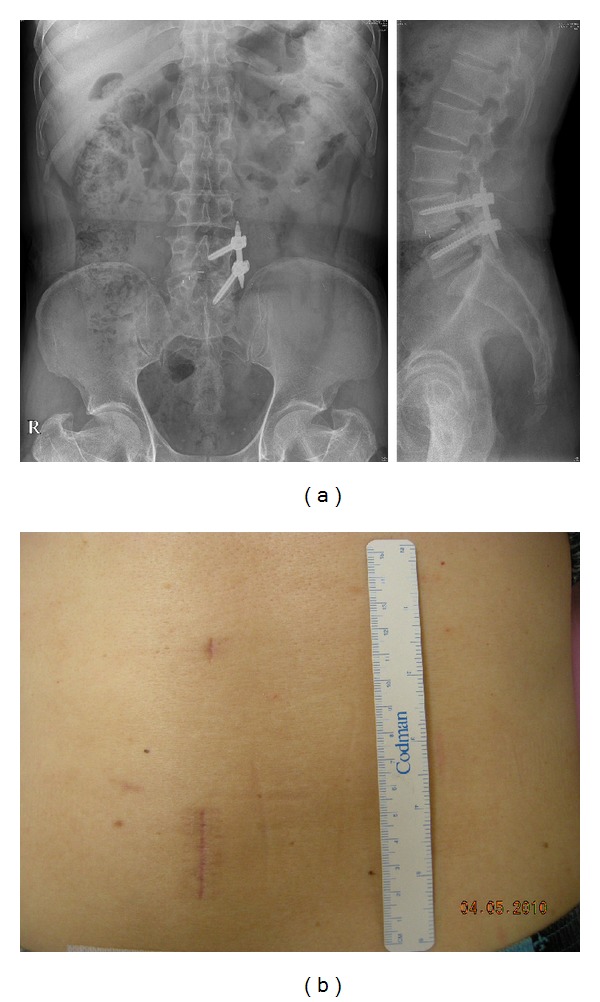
Minimally invasive transforaminal lumbar interbody fusion with unilateral percutaneous pedicle screw fixation. X-ray (a) and final skin incision (b). An ipsilateral incision for the tubular retractor, an ipsilateral percutaneous pedicle screw system, and an upper incision for rod insertion (b).
